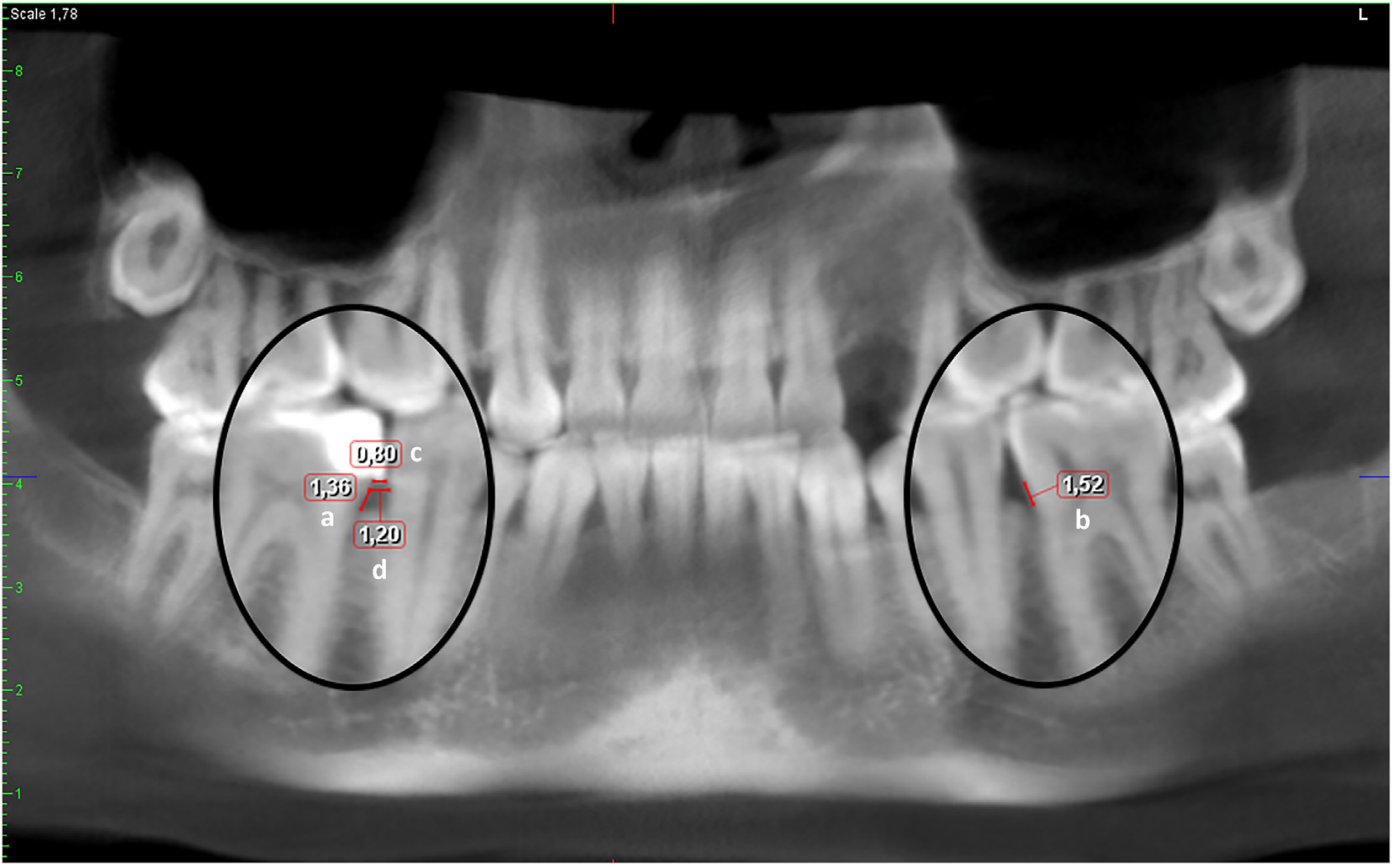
CBCT reformatted panoramic image showing the measurement of a) the amount of bone loss adjacent to the overhanging approximal restoration, b) the amount of bone loss on the same surface of the contralateral control tooth, c) the amount of the overhang, and d) the distance between the CEJs of the relevant and the adjacent teeth. The size of the overhang was calculated by using the formula (c/d) *×* 100. In this case, the size of the overhang occupying 66% ((0.80/1.20)*×*100) was classified as large (occupying more than 51% of the interproximal space).
